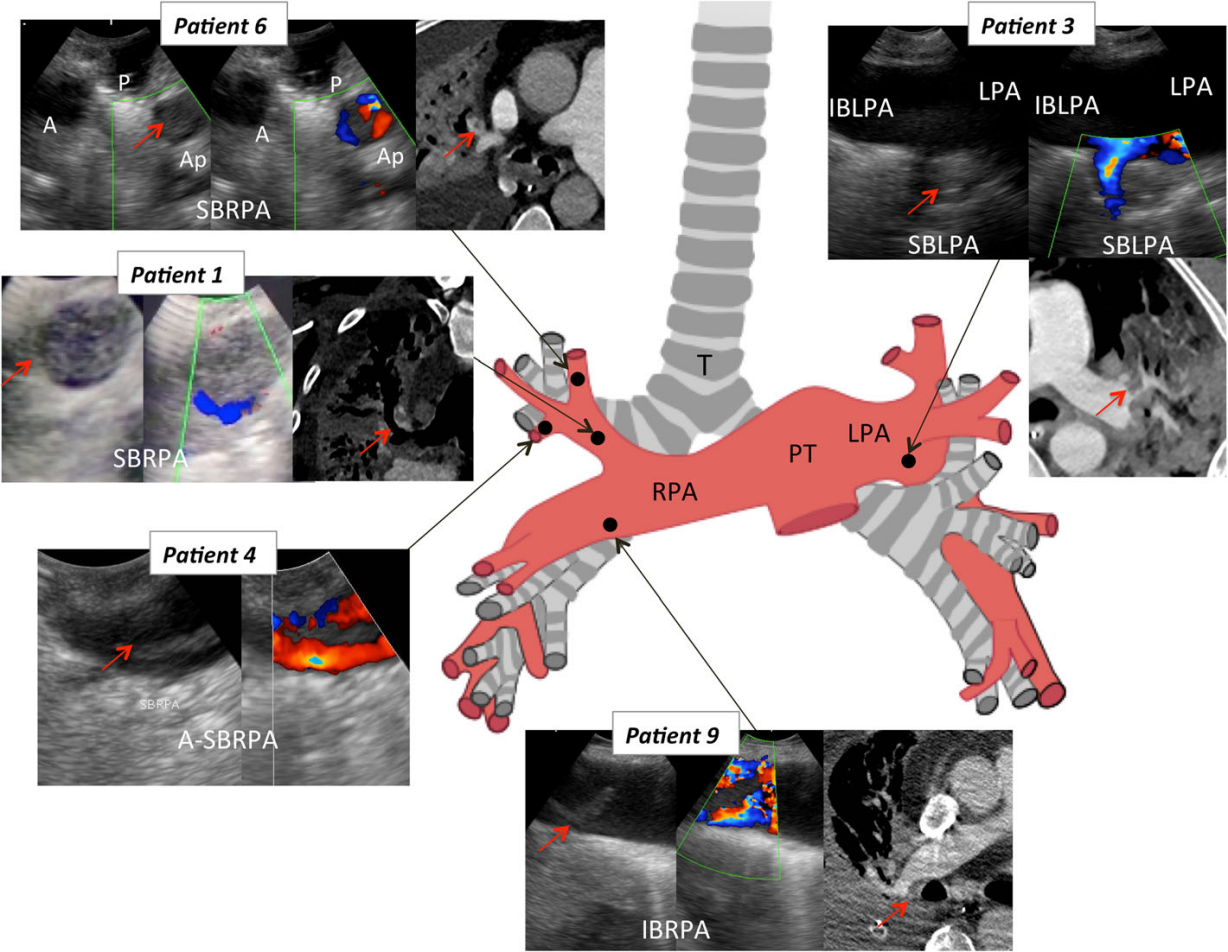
Endobronchial ultrasound and computed tomography pulmonary angiography correspondence in the five patients with pulmonary embolism. Red arrows indicate the presence of thrombus in pulmonary arteries. SBRPA, superior bronchial right pulmonary artery; A-SBRPA, anterior segment of the SBRPA; P-SBRPA, posterior segment of the SBRPA; A-SBRPA, apical segment of the SBRPA; LPA, left pulmonary artery; IBLPA, inferior bronchial left pulmonary artery; SBLPA, superior bronchial left pulmonary artery; T, trachea
